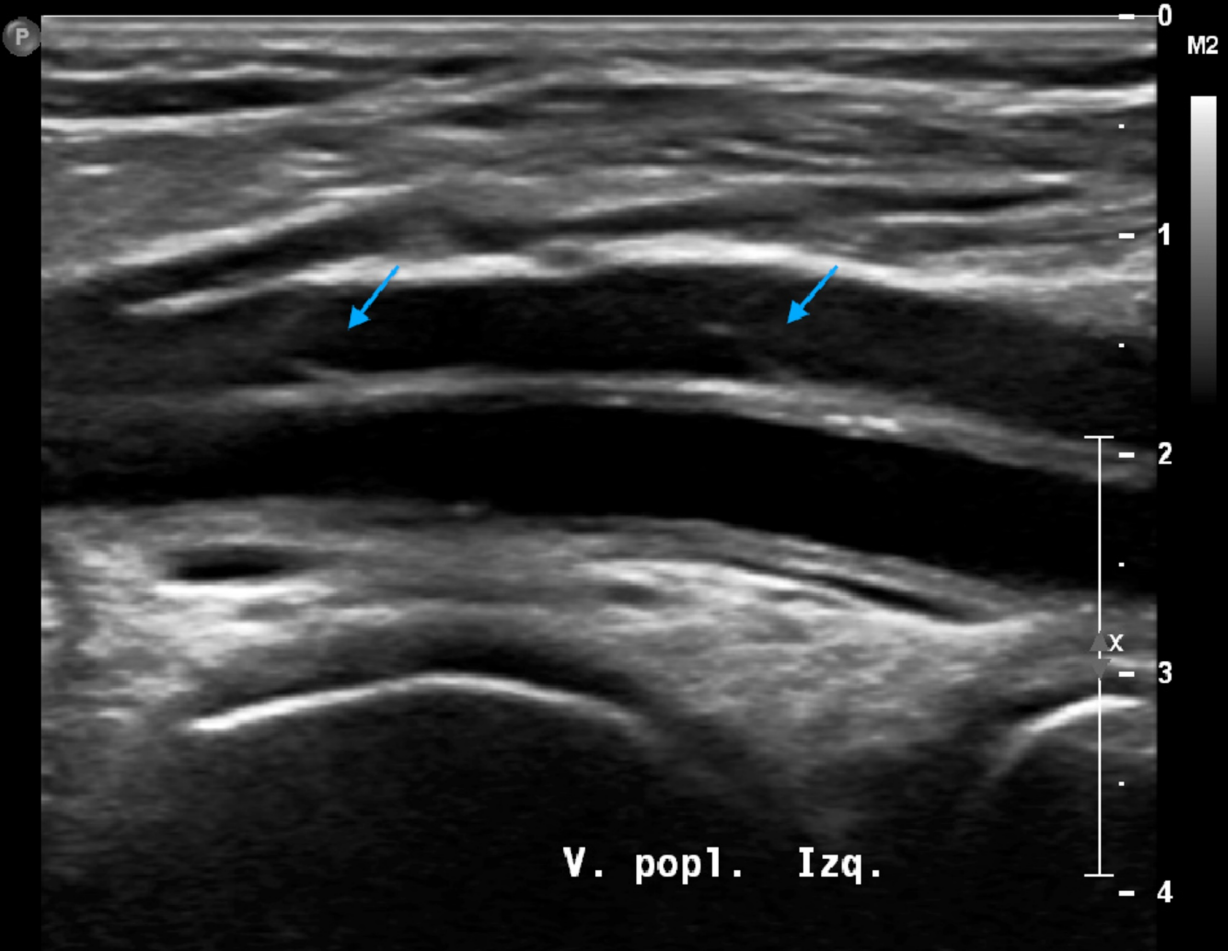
Sonogram of the left popliteal vein taken 13 days after the first one A clean venous lumen with normal valvular function can be seen (blue arrows).
